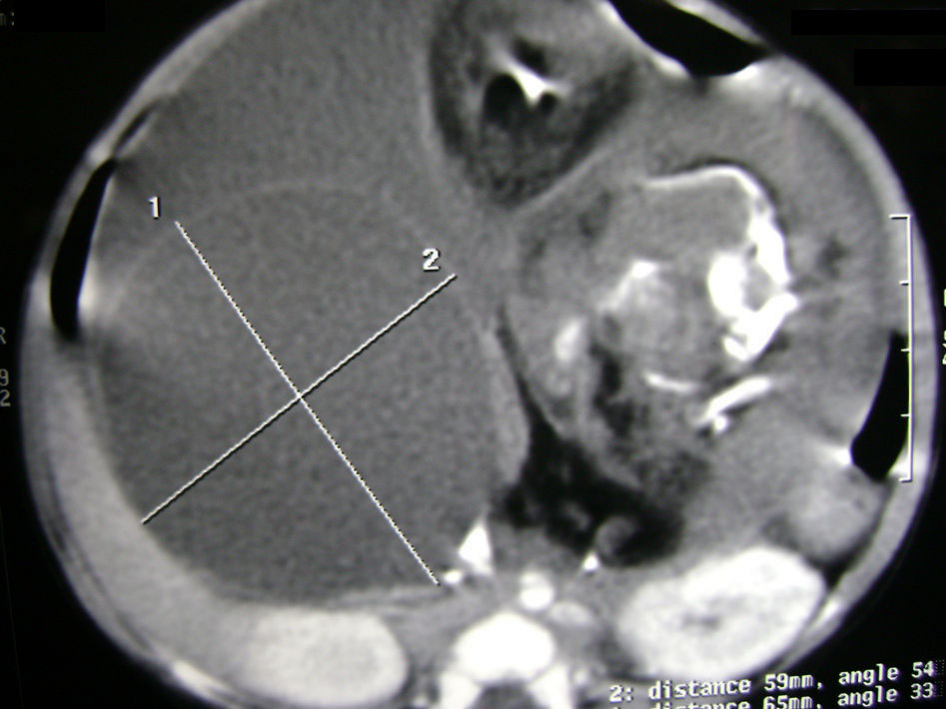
Abdominal computed tomography of the fetus with a large encapsulated peritoneal cavity mass and mature vertebral skeleton.
